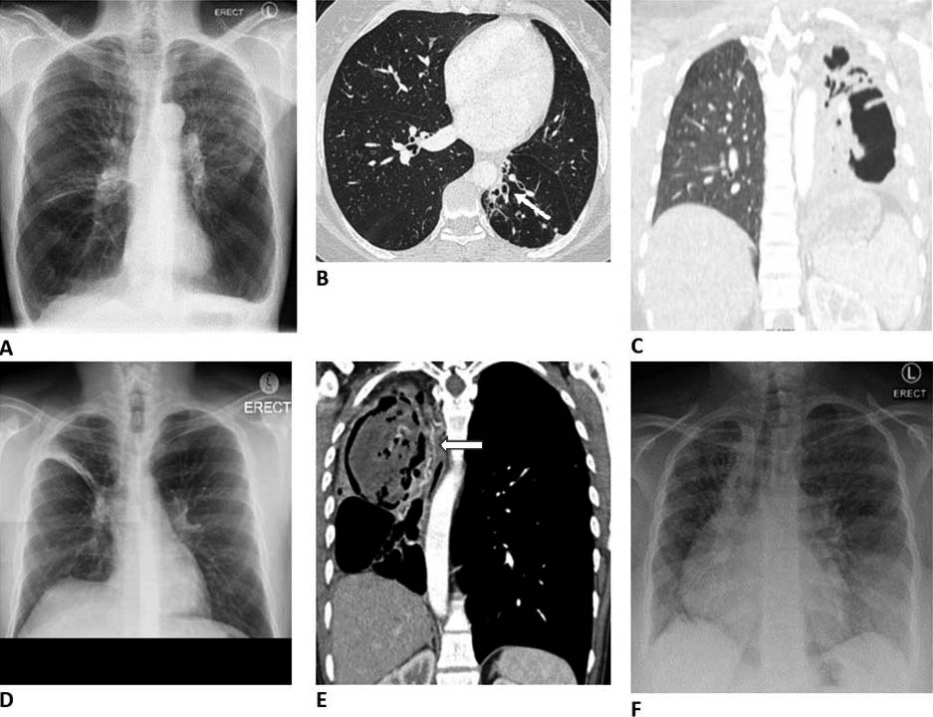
Images and case descriptions corresponding to clinical patterns in post-TB lung disease (see Table 2): A) TOPD in 58-year-old male with three previous episodes of TB, a 10 pack-year history of smoking, and a FEV_1_/FVC of 28%. B) Focal bronchiectasis of the left lower lobe (arrow) in a 38-year-old female with two previous episodes of TB. C) Complete destruction of the left lung in a 31-year-old female, after two episodes of TB. D) Residual fibrotic band in the right upper lobe of a 46-year-old male, with one episode of TB 5 years prior. E) Right upper lobe cavity containing an aspergilloma in a 40-year-old female with recurrent haemoptysis after four previous episodes of TB. Note the dilated bronchial arteries (arrowhead). F) Chest X-ray of a 29-year-old female with pulmonary hypertension after two episodes of drug-resistant TB. Her mean pulmonary artery pressure was 65 mmHg at right heart catheterisation, and she had features of both TOPD and bronchiectasis. Images courtesy of B. Allwood. TOPD = TB-associated obstructive lung disease; FEV_1_ = forced expiratory volume in 1 sec; FVC = forced vital capacity.
